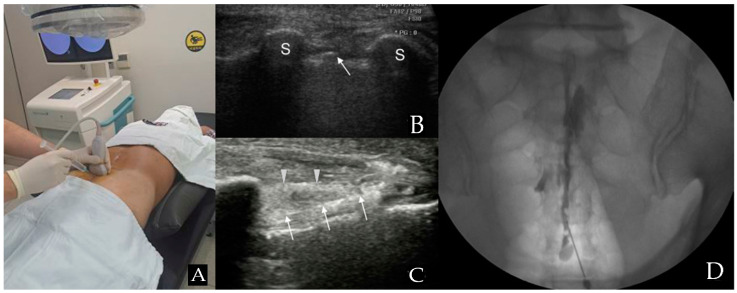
Combined ultrasound and fluoroscopy-guided caudal epidural steroid injection. (A) Utilizing ultrasound transverse view to insert the spinal needle in the appropriate location. (B) Ultrasound image seen from the probe in (A) position. The arrow points to the caudal epidural needle, which is located between the two sacral cornua, indicated by S (hyperechoic reversed U shape) in the image. (C) A view along the longitudinal axis displays the needle within the caudal epidural space. The arrowhead is pointing to the sacrococcygeal ligament, while the arrow points to the caudal epidural needle. (D) An anteroposterior X-ray reveals the contrast medium’s epidural spread following the administration of a caudal epidural injection.
